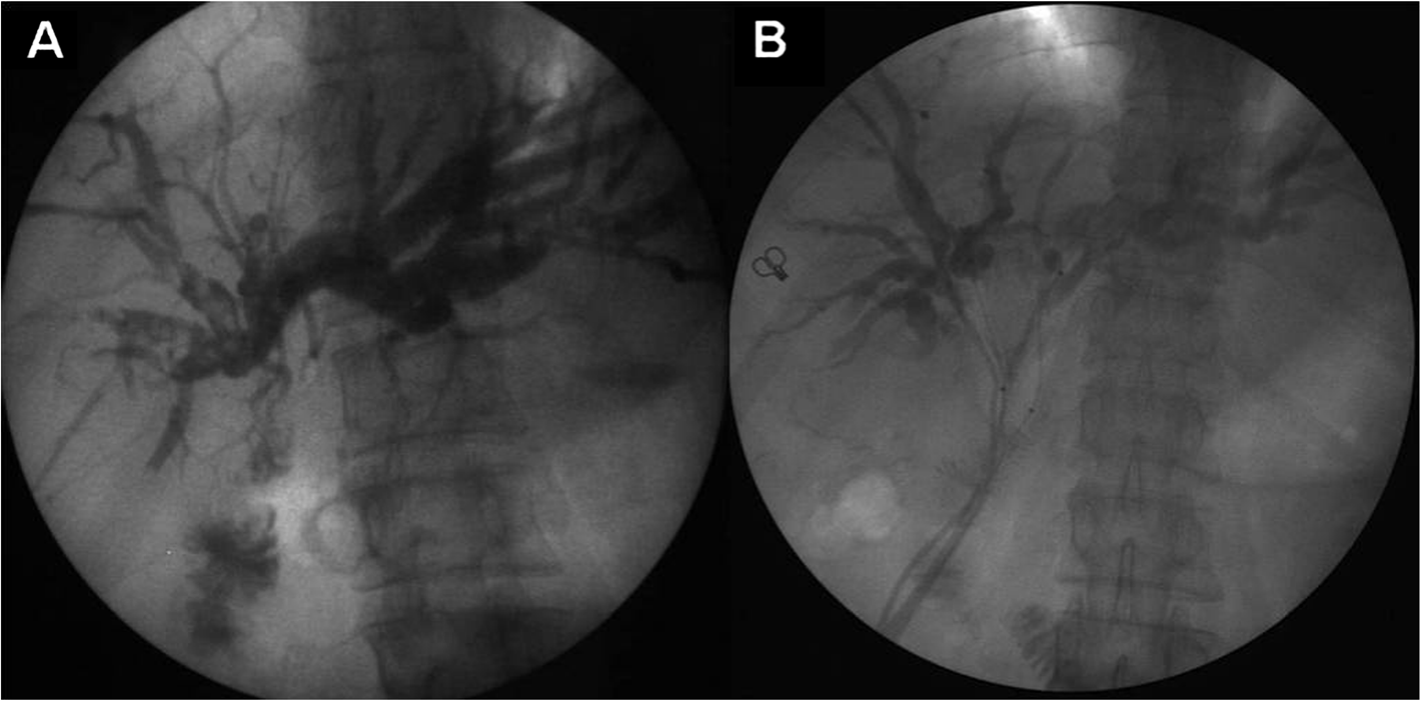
Images of biliary system before and after HJPBD. A: Before HJPBD, the X-ray photography with cholangiography showed that the lesions located at the common hepatic duct stump with the ceiling of the confluence was destroyed; B: Two months after HJPBD, the X-ray photography with cholangiography showed that the two balloons were still at their positions, both passing through the anastomotic stoma with one end in the enteric cavity and the other stretching into the left or right hepatic bile duct. HJPBD: progressive balloon dilation following hepaticojejunostomy.
